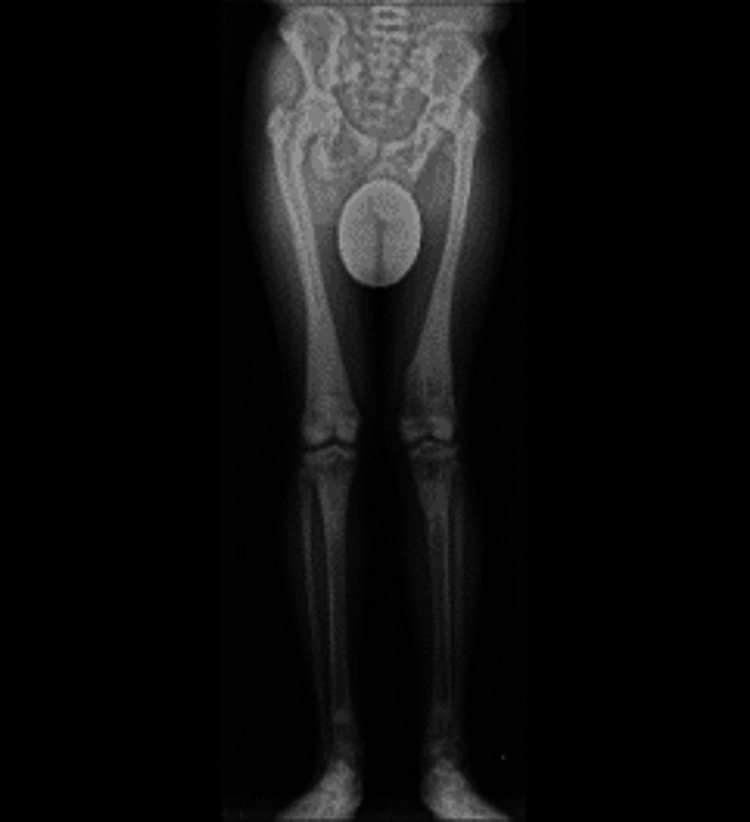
Preoperative full-leg X-ray image
